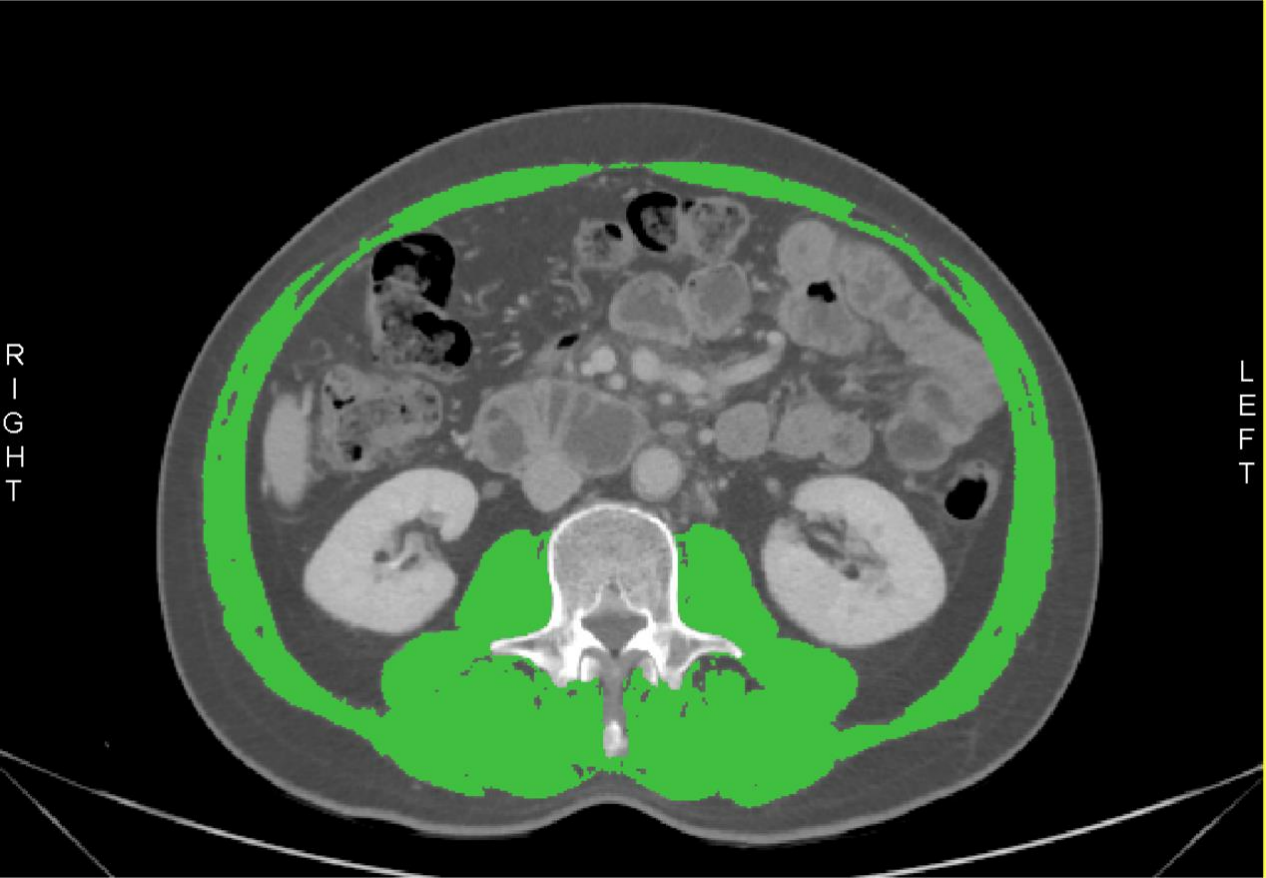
Skeletal muscle assessment using computed tomography.Muscle segmentation was conducted on the cross-sectional image at the L3 vertebra, using a threshold range of −29 to +150 HU (shown in green). This included the psoas major, erector spinae, quadratus lumborum, transversus abdominus, as well as the external and internal oblique muscles, and the rectus abdominus.
